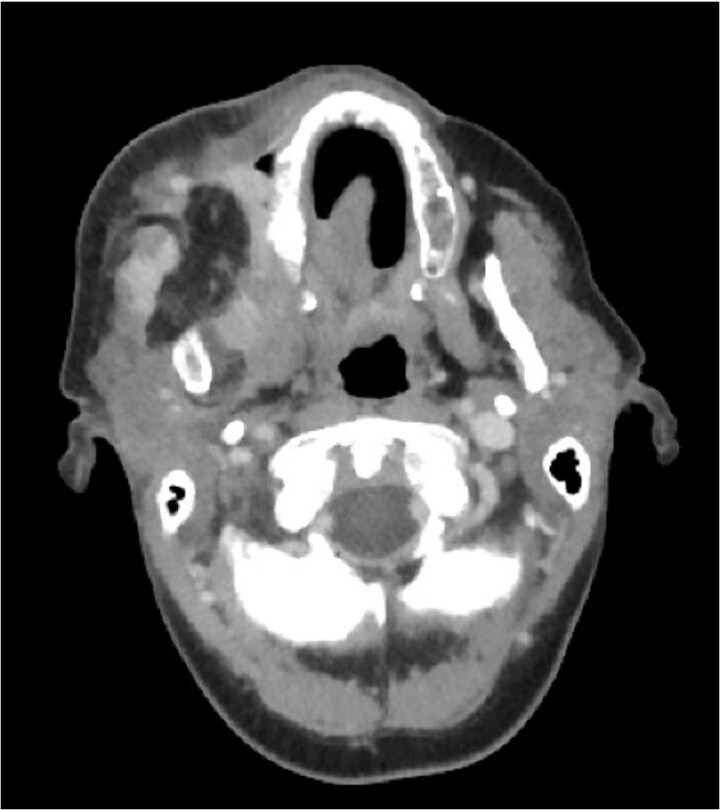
CT neck with contrast 2 months post-surgery: Focus of enhancement deep to resection cavity within pterygoid musculature measuring 1.3 × 1.1 cm with associated radiotracer uptake.
